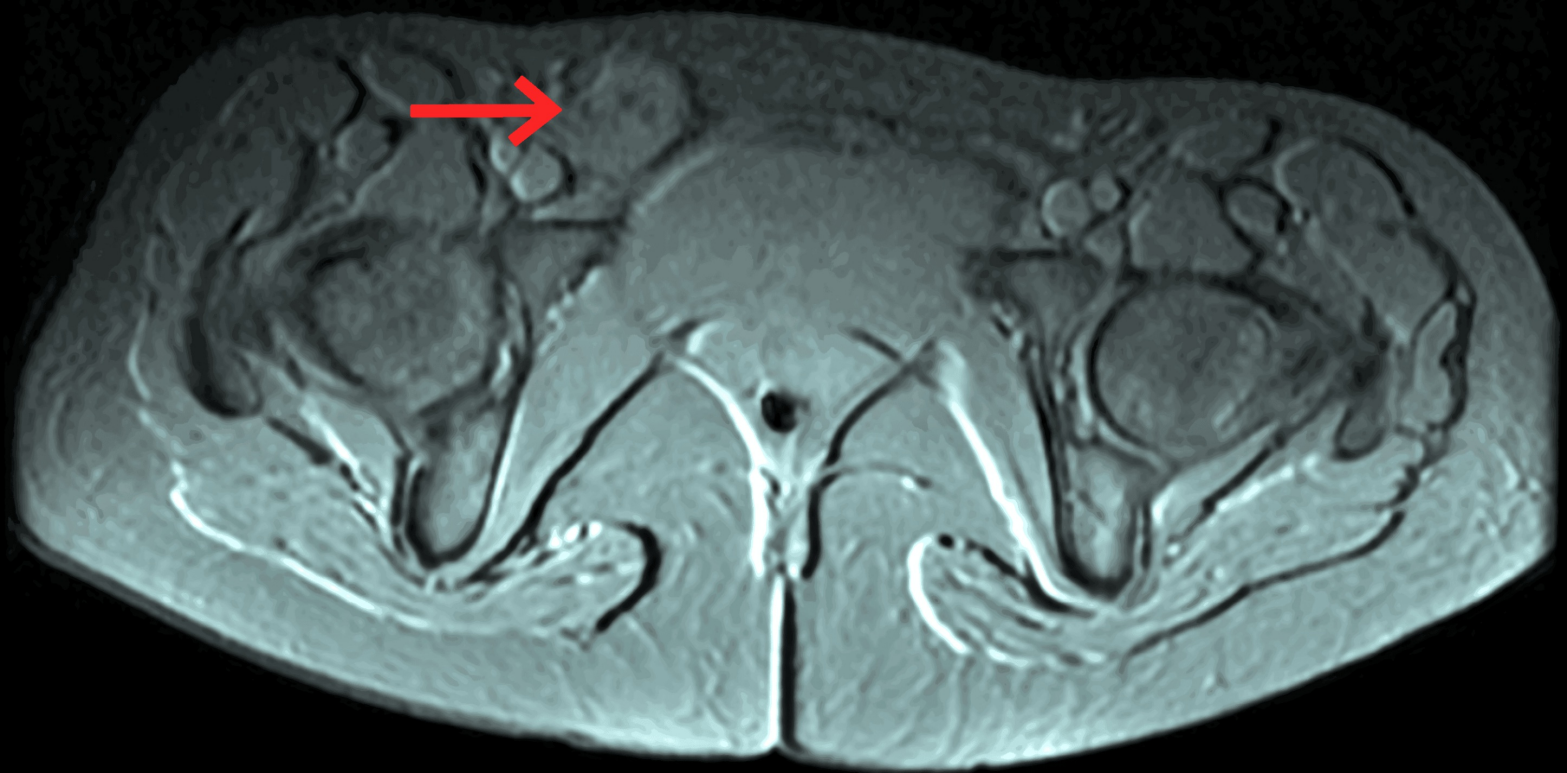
Pelvic MRI showing a well-defined round mass approximately 2.4 x 2.4 x 2.2 cm in size extending from the right inguinal canal into the subcutaneous plane of the right lateral pubic area revealing few areas of blooming on the T2* GRE sequence MRI: Magnetic resonance imaging; T2* GRE sequence: T2-star gradient echo sequence
